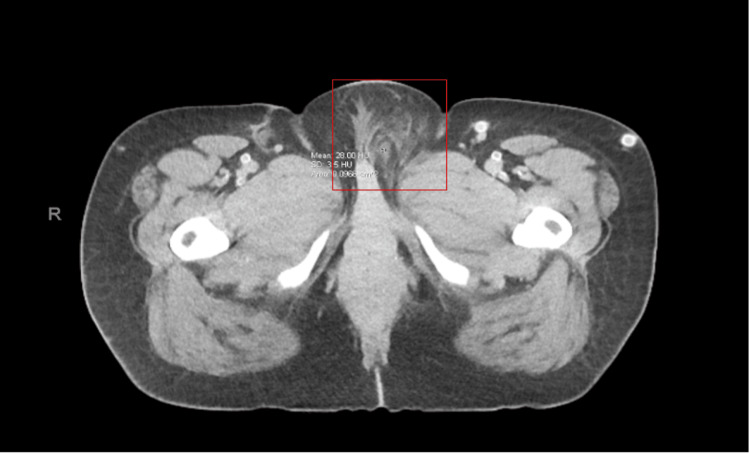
Contrast CT of the abdomen and pelvis on the day of admission Left labial cellulitis (red box) with no soft tissue gas or abscess collections
